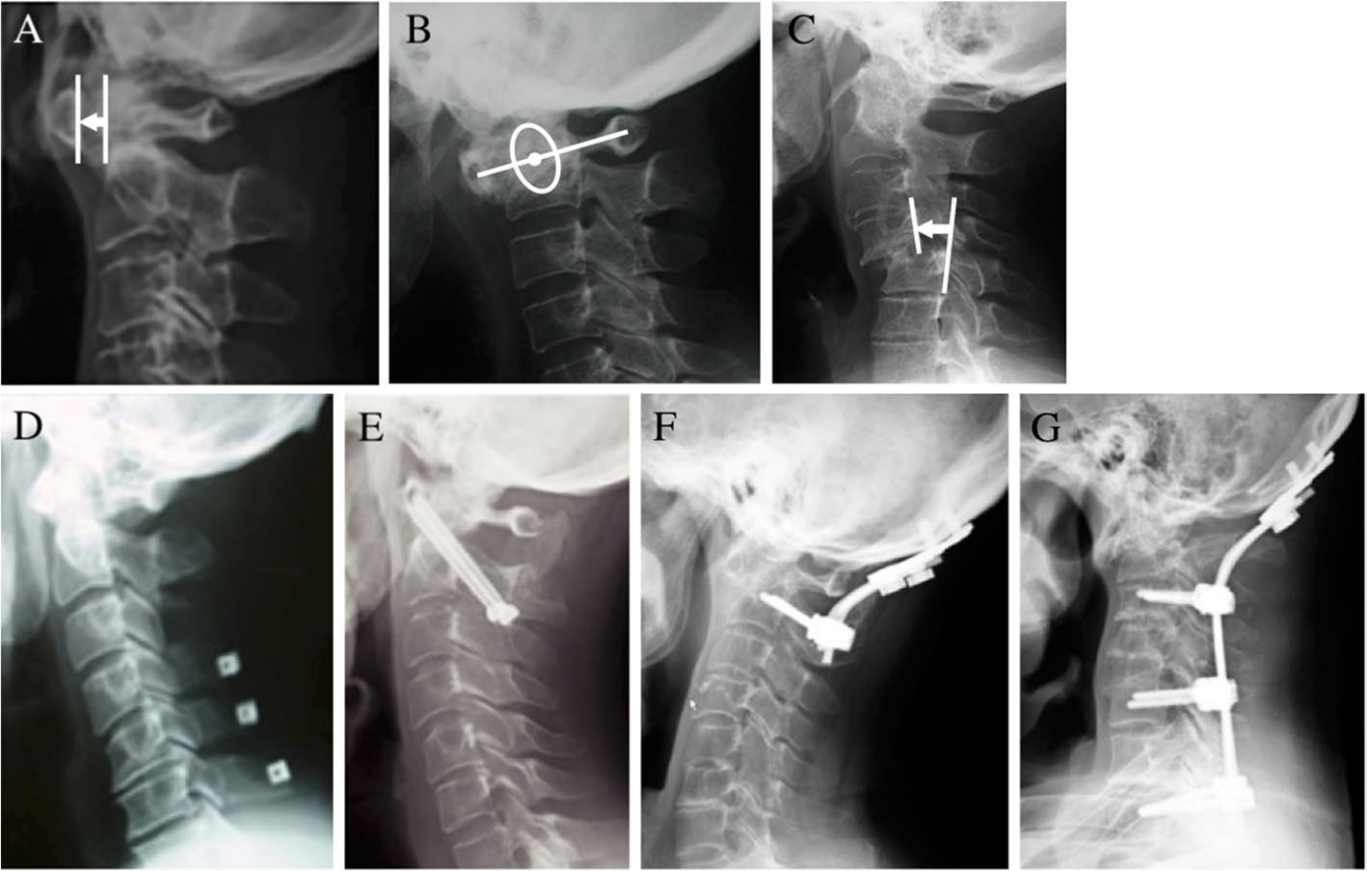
Cervical lesions associated with RA and surgical procedures for individual cervical lesions. A Atlantoaxial subluxation (AAS) was defined as an expansion of the atlantodental interval over 3 mm (between the white lines) at the flexed position on X-ray lateral view. B Vertical subluxation (VS) was defined as a shortening of the Ranawat C1–C2 index to less than 15 mm for men and 13 mm for women. The measurement of the Ranawat index was made from the center of the pedicles of the axis (white circle and dot) to a line connecting the midpoint of the anterior and posterior arches of the atlas (white line). C Subaxial subluxation (SAS) was diagnosed as migration of the superior vertebra compared to the inferior vertebra over 3 mm. Migration distance was measured between posterior walls of adjacent vertebrae (between the lines). D–G Four types of surgical procedures were performed for cervical spine lesions in patients with RA. Laminoplasty was performed for myelopathy in the stable cervical spine (D). Fixative procedures were chosen depending on how unstable the cervical spine was: atlantoaxial fusion for unstable AAS (E), occipital-cervical fusion for VS with or without AAS (F), and occipital/thoracic fusion for SAS that included AAS and/or VS (G)
